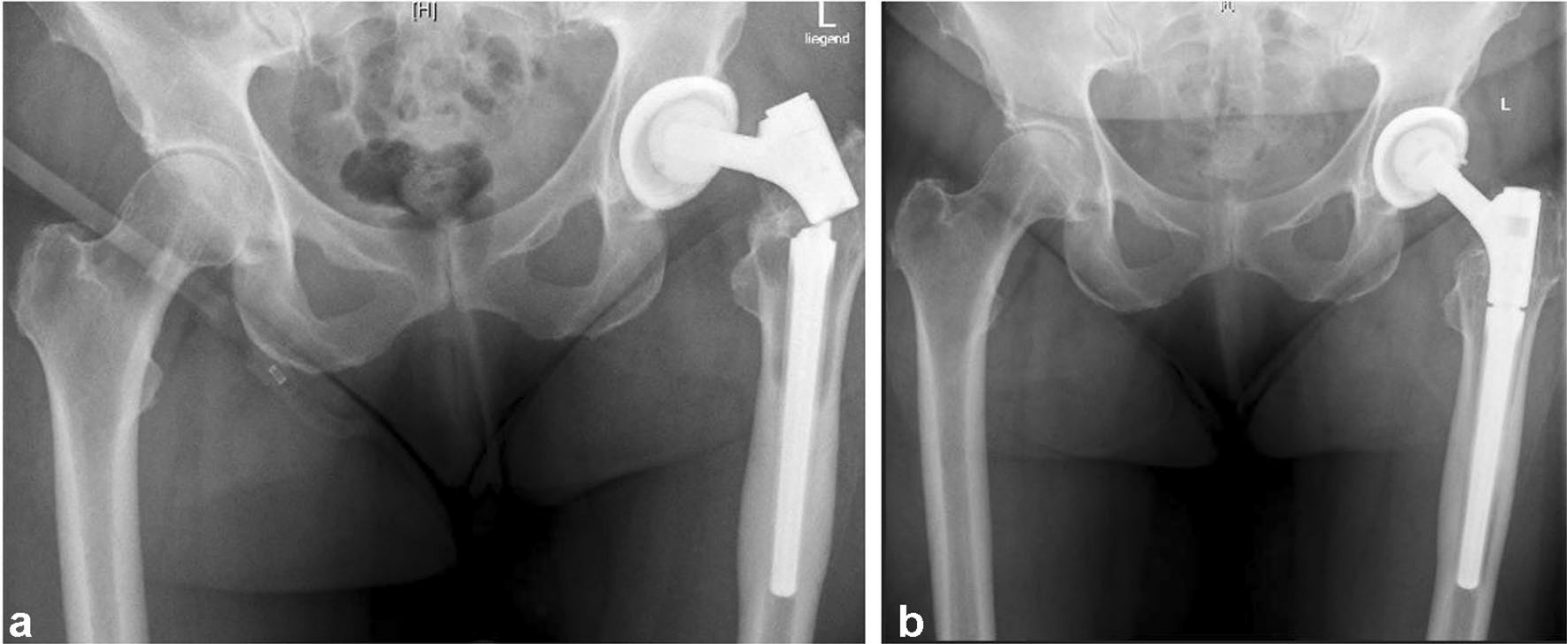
Pelvic radiograph demonstrating implant fracture at the junction between the neck component and the stem 5.3 years after implantation (left side). A 16 × 140 mm stem with a short-length neck segment and a lateralized offset was used in this patient. Notice the extensive osteolysis around the stem in the proximal Gruen zones. Femoral revision was performed with the use of a 17 × 140 mm MRP-Titan stem and a medium-size neck segment with lateralized offset. Follow-up radiograph 7 years after stem re-revision shows full osteointegration of the stem and complete reossification of the osteolysis (right side)
